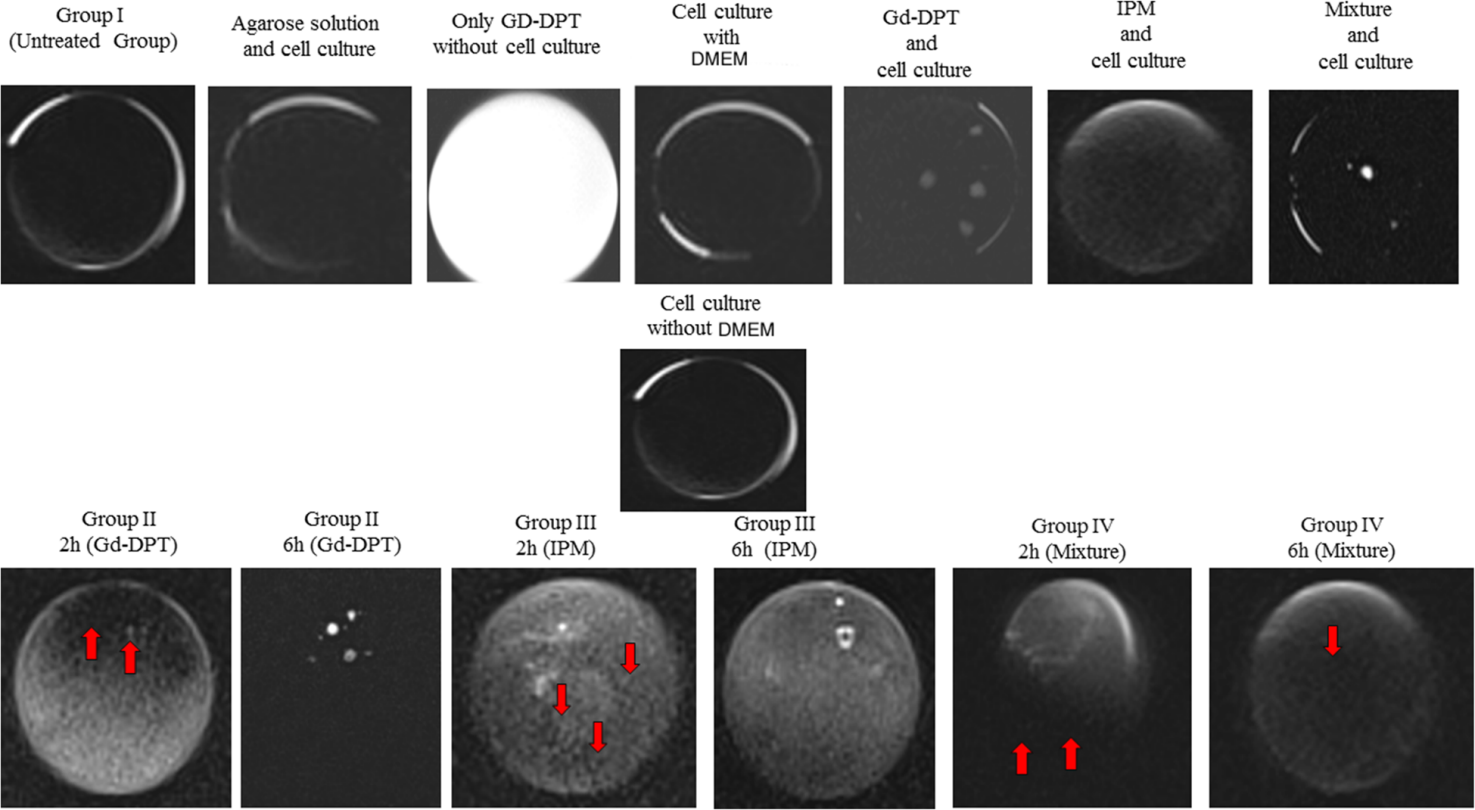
Evaluation of chondrocytes through 3-tesla magnetic resonance imaging
